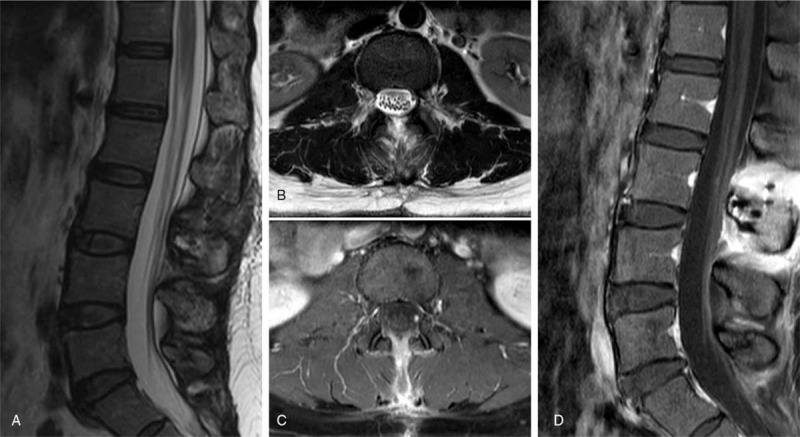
Postoperative spinal MRI (1-year follow-up) showed no evidence of tumor recurrence. (A) Sagittal T2-weighted image (WI); (B) axial T2-WI; (C) axial contrast-enhanced image; (D) sagittal contrast-enhanced image. MRI = magnetic resonance imaging.
